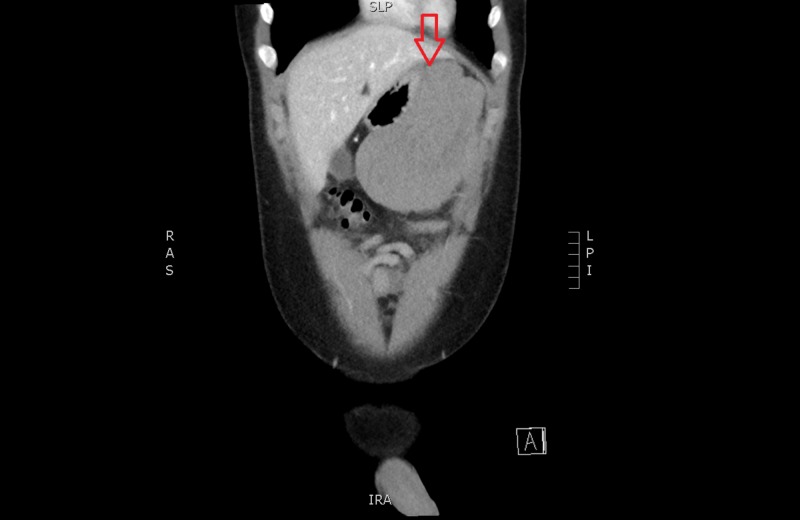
Computed tomography of the abdomen (coronal) showing a heterogeneous mass from the greater curvature of the stomach (see red arrow)
